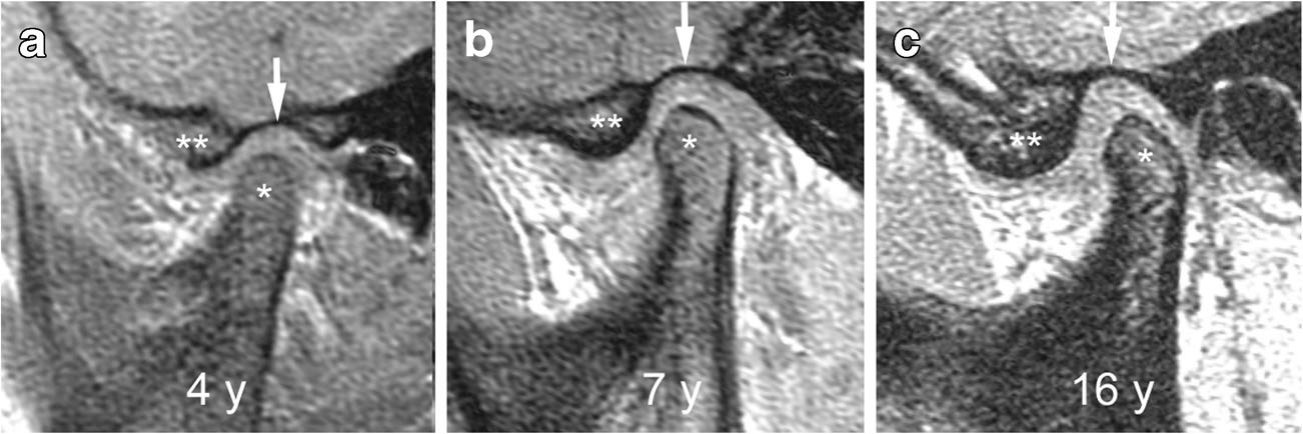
Normal shape of mandibular condyle (grade 0) and temporal bone on sagittal oblique T1-weighted gradient echo images (TR/TE 300/4.2 ms, flip angle 80°, without fat saturation). a-c Images from three different children show the changing configuration of the mandibular condyle (*), the articular eminence () and the glenoid fossa (arrows) among a 4-year-old (a), a 7-year-old (b) and a 16-year-old (c). In a 4-year-old child (a) the superior articular contour is round with a straight condylar neck. With increasing age and growth, the condylar neck gains an anterior tilt and the head appears more angular, with less rounding of the anterior-superior joint surface. The articular eminence gets larger resulting in a deeper glenoid fossa
